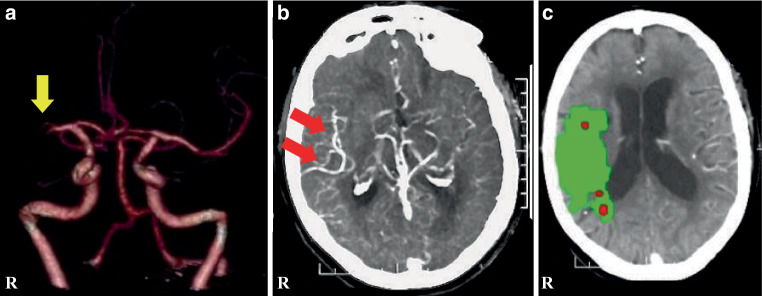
Representative case presentation; a patient had right middle cerebral artery proximal occlusion. Each image was performed at 2 h after stroke onset. a 3D-CT angiography; *yellow arrow* shows right middle cerebral artery proximal occlusion. b Dynamic CT angiography; *red arrows* show vessels reconstituted by collateral flow. c CT perfusion image; *green area* shows hypoperfusion lesion (90 ml) and *red area* shows ischemic core lesion (6 ml). Mismatch volume (penumbra lesion) was 84 ml. CT perfusion collateral index: (delay time > 6 s lesion 21 ml/delay time > 2 s lesion 124 ml) × 100 = 17
